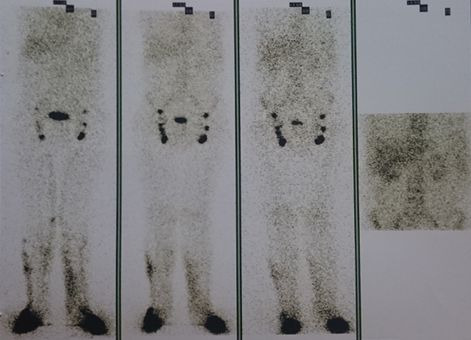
Lymphoscintigraphy showing bilateral dermal backflow.
